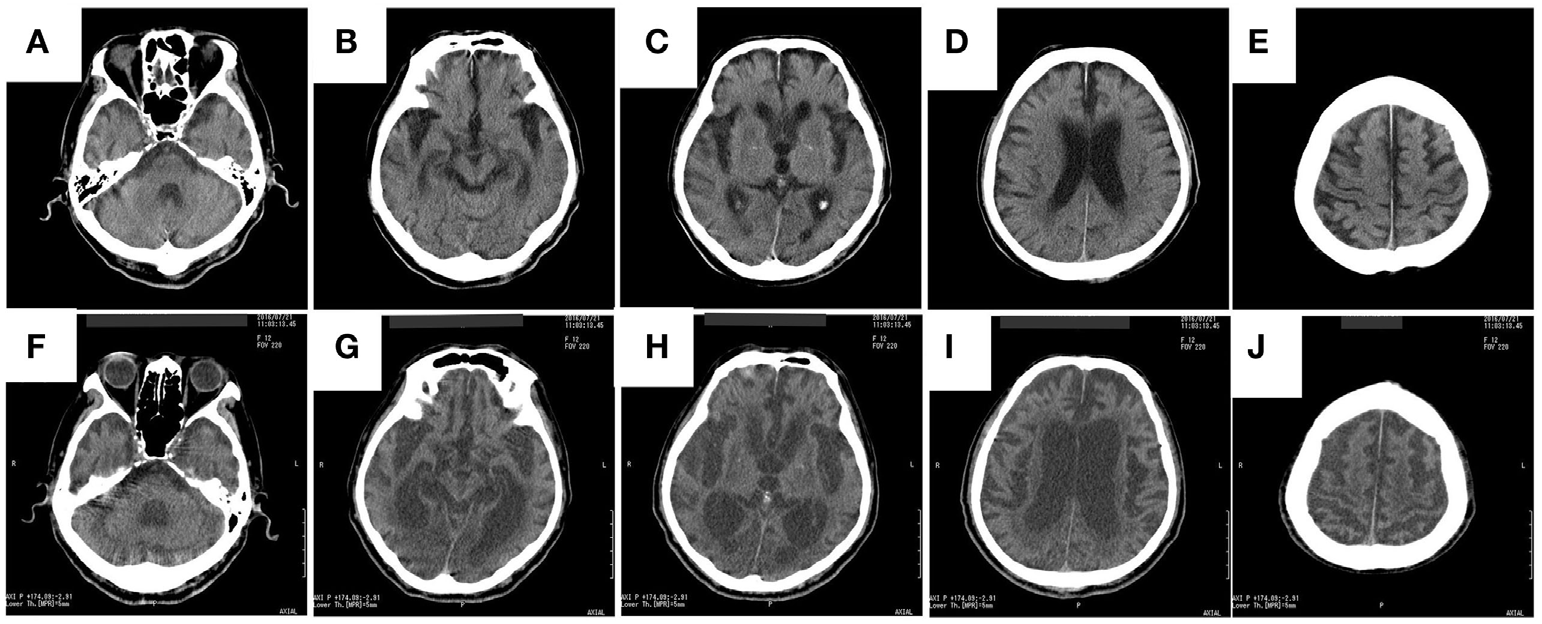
The rapid progress of brain atrophy in the MSA patient after developing hypoxic encephalopathy axial CT images of the patient acquired in September 2015 (A–E) and in July 2016 (F–J) are shown. MSA, multiple system atrophy; CT, computed tomography.
